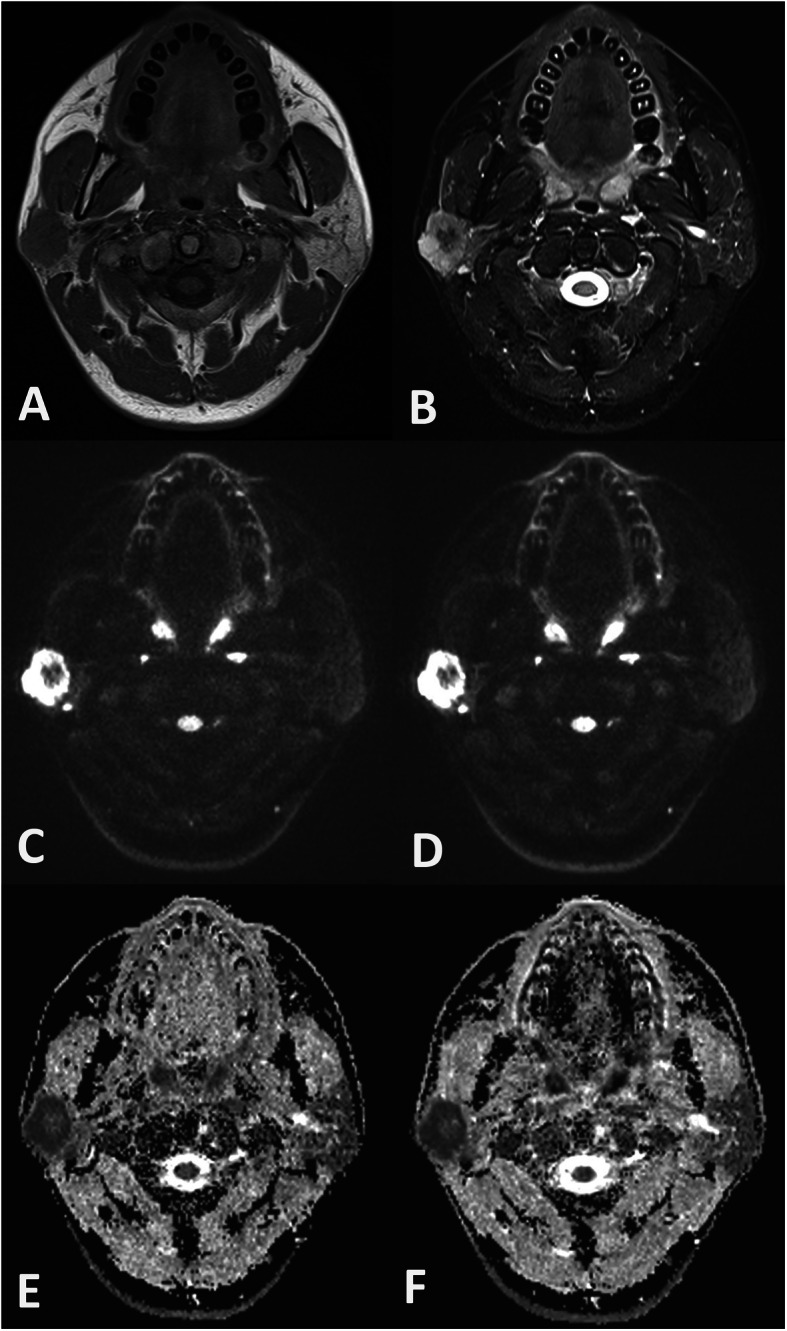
Example of SMS-RESOLVE DWI, RESOLVE DWI, and corresponding ADC maps of a 29-year-old patient with a lymphoepithelioma in the right parotid gland. The tumor showed isointensity on T1-weighted image (a), hyperintensity on T2-weighted image (b), and peripheral hyperintensity and central hypointensity on axial DWI (c, SMS-RESOLVE DWI; d, RESOLVE DWI). Image quality scores were similar for SMS-RESOLVE DWI and conventional RESOLVE DWI. The ADC value of the tumor derived from SMS-RESOLVE DWI (e) and RESOLVE DWI (f) were 0.54 × 10^− 3^ mm^2^/s and 0.52× 10^− 3^ mm^2^/s, respectively
